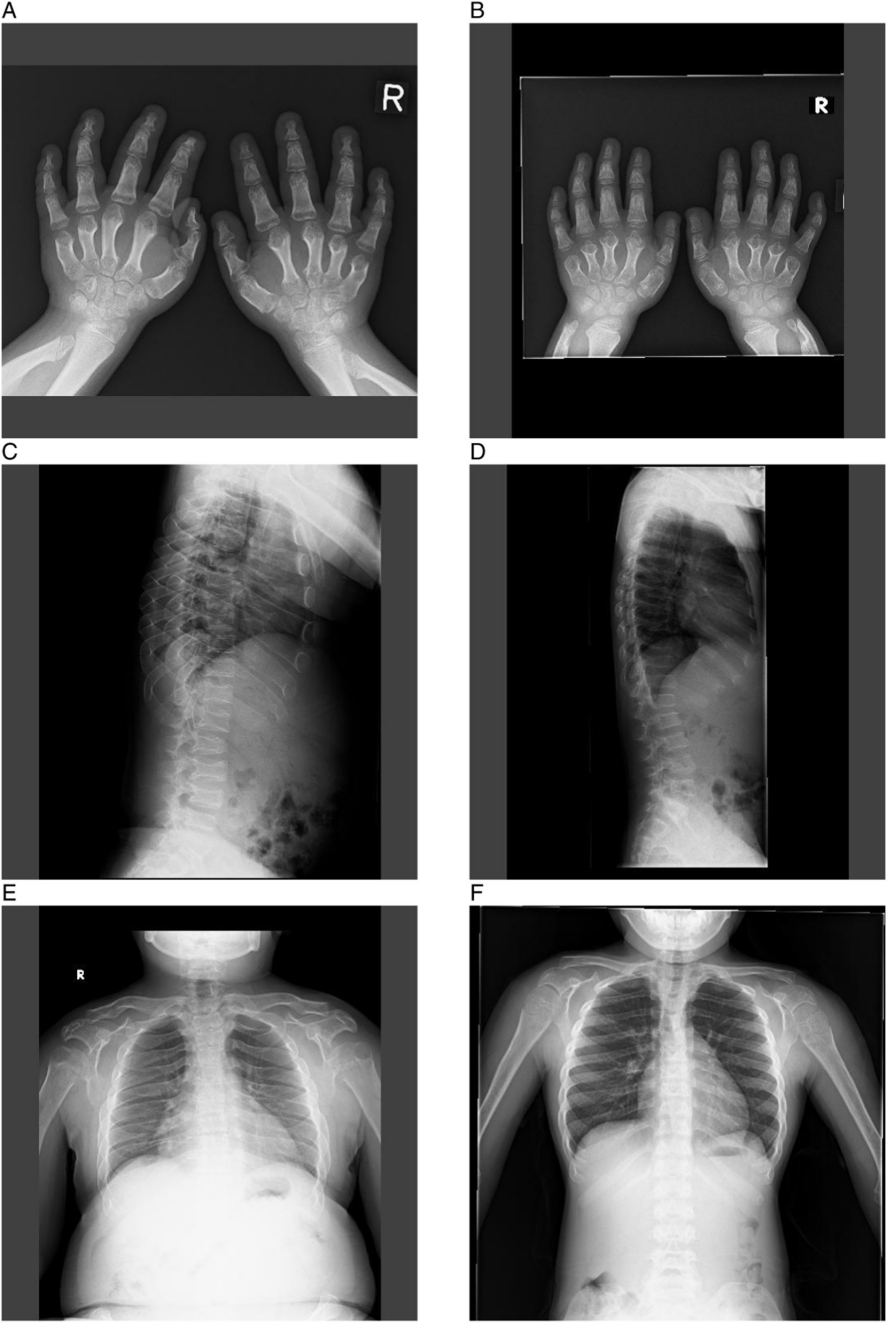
Radiographs of hands and spinal column of Sibling 1 (A, C, E) and Sibling 2 (B, D, F) at 10.2 and 9.7 years, respectively, after starting enzyme replacement therapy.
